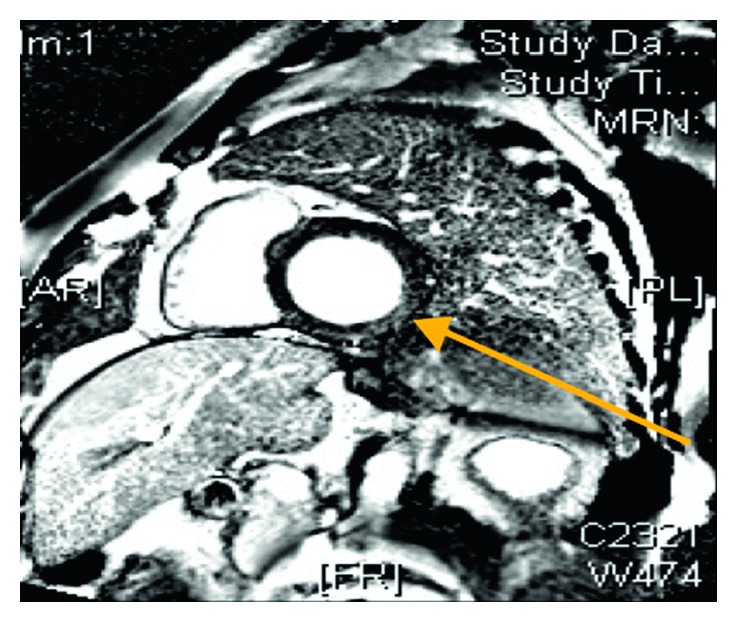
Cardiac MRI inversion recovery images after contrast injection revealing mild mid-wall enhancement in the basal inferior and inferolateral walls consistent with myocarditis (arrow).
